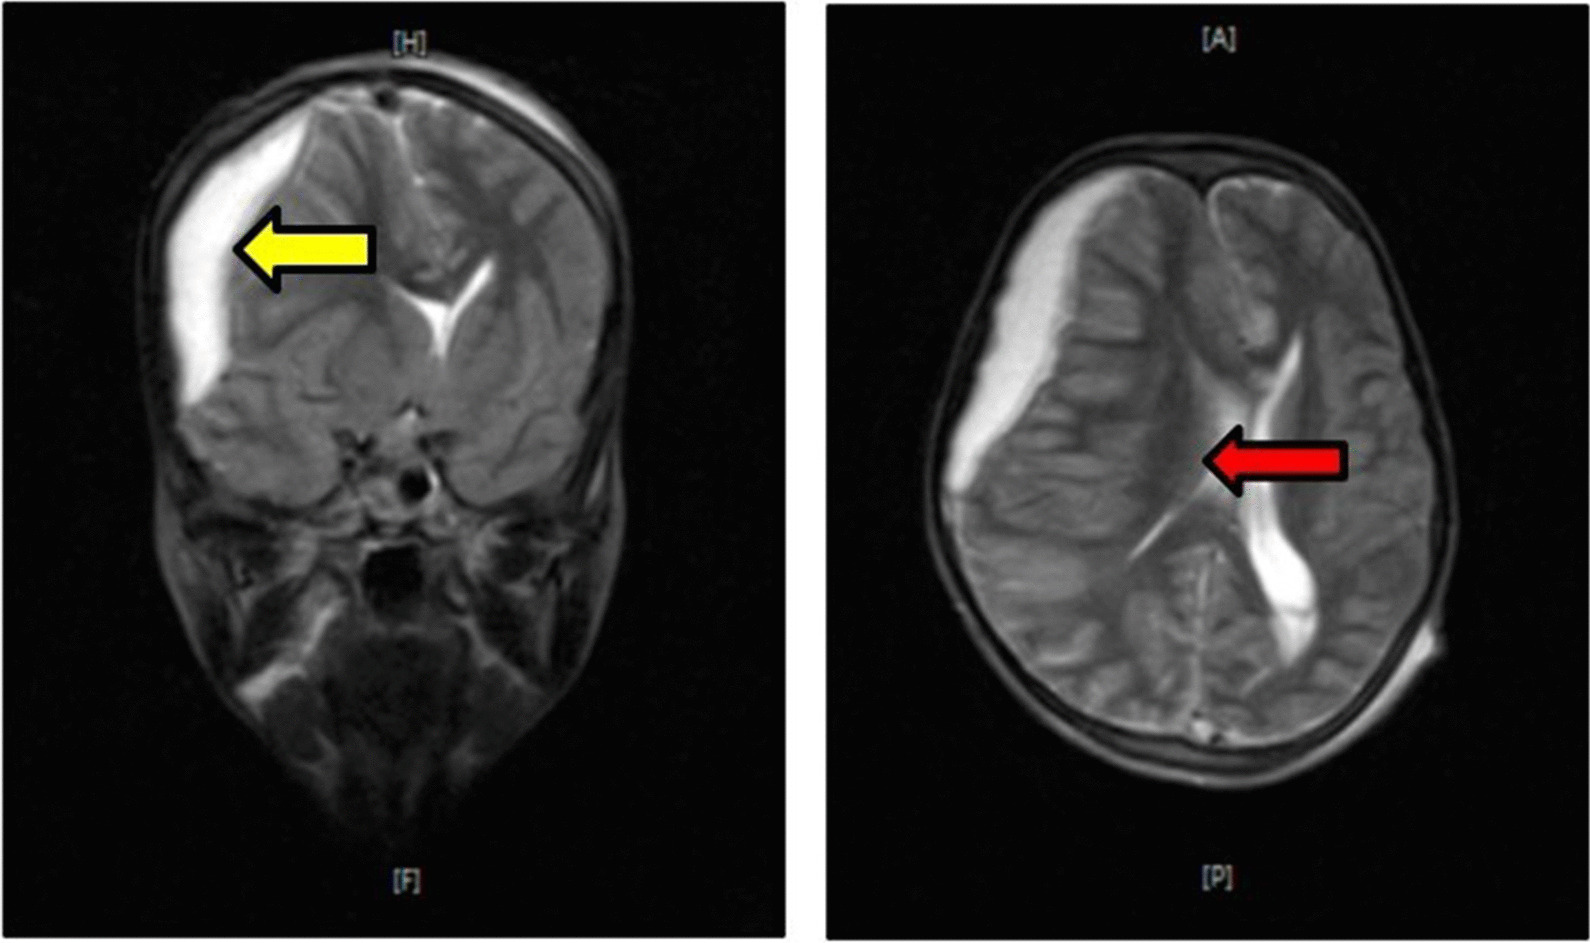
MRI images of the patient. Figure clearly shows a right fronto-temporal-parietal subdural empyema (yellow arrow) effacing the adjacent cortical sulci and compressing the right lateral ventricle, midline shift to the left (red arrow)
